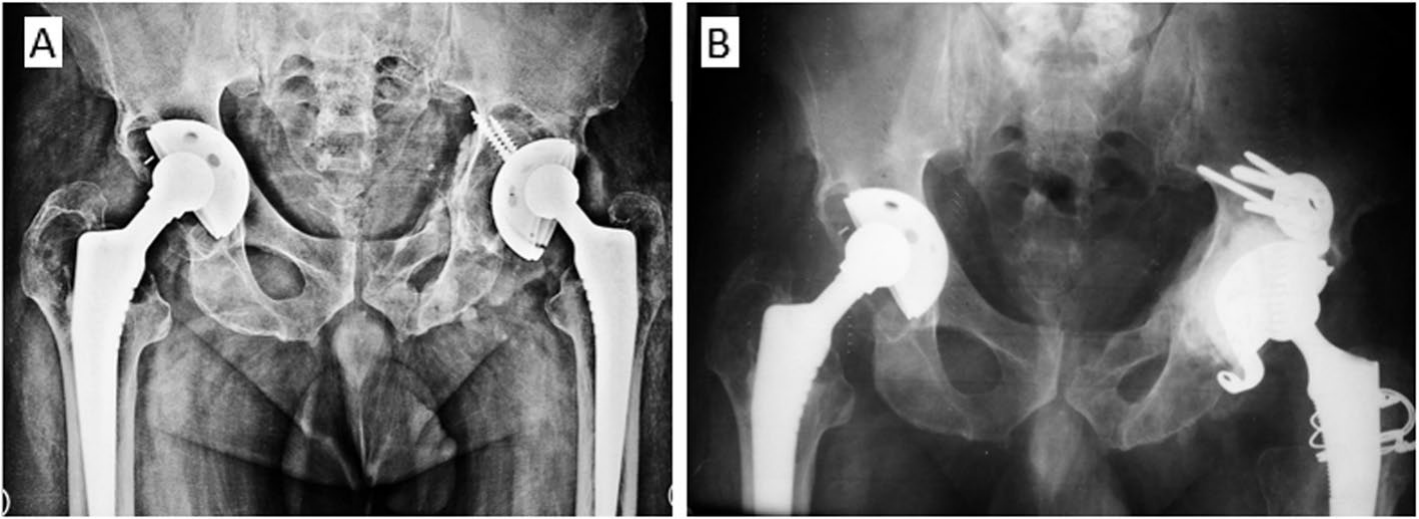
A preoperative x-rays for loose left acetabular cup with massive osteolysis Paprosky Grade IIIB and fracture pubic rami that indicated impaction graft and KE ring. B postoperative x rays with the full incorporation of the impaction graft, healing of pelvic fractures and stable cemented Novae stick cup
